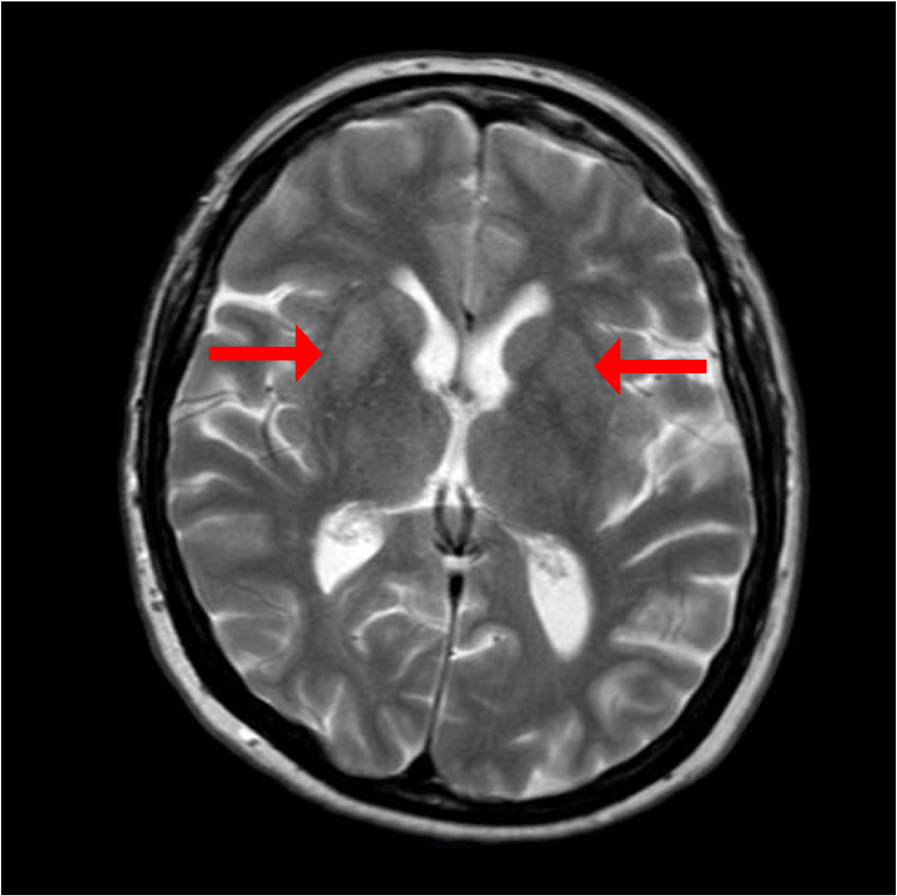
Magnetic resonance image of the patient during admission. T2-weighted magnetic resonance image showing bilateral symmetrically increased signal intensity in the basal ganglia (*red arrows*)
